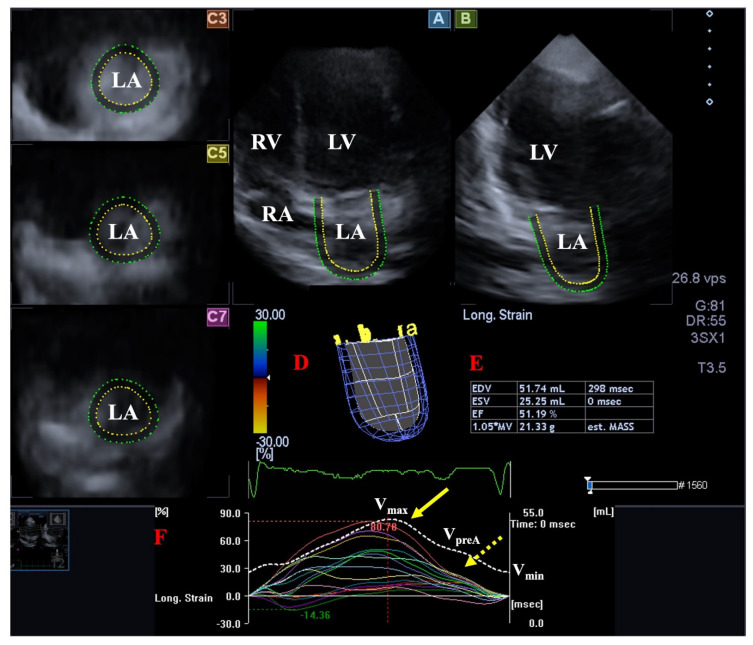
Evaluation of the left atrium (LA) by three-dimensional (3D) speckle-tracking echocardiography. Following acquisition of a 3D echocardiographic dataset and by using a dedicated vendor-provided software, several views are produced automatically: Longitudinal apical four-chamber (A) and two-chamber (B) views, together with short-axis views at the basal (C3), midatrial (C5), and superior (C7) LA regions, are demonstrated. A virtual 3D LA cast (D), together with calculated LA volumes (E) and time–LA global (white curve) and segmental (colored curves) longitudinal (F) strain curves with a time–LA volume change curve (dashed white curve), are also demonstrated. The yellow arrow represents peak LA strains, whereas the yellow dashed arrow represents LA strains at atrial contraction. Abbreviations: LV = left ventricle, LA = left atrium, RV = right ventricle, RA = right atrium, EDV = end-diastolic volume, ESV = end-systolic volume, EF = ejection fraction, MASS = LA muscle mass, Vmax = minimum end-systolic LA volume, VpreA = early diastolic LA volume before atrial contraction, Vmin = end-diastolic minimum LA volume.
